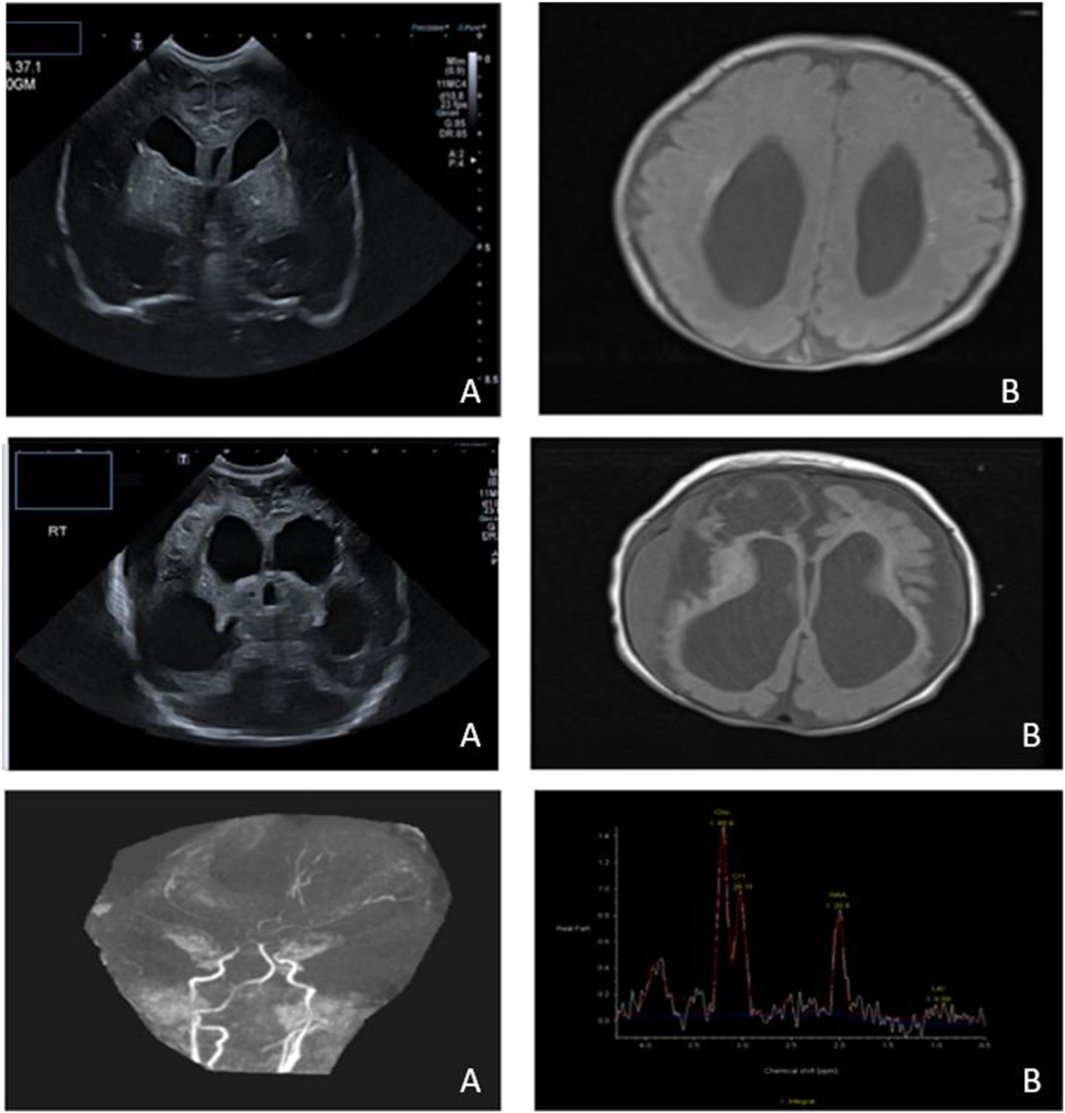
Serial neuroimaging. *Top panel:* (A) cranial ultrasound image on day 1 of life showing ventriculomegaly with colpocephaly (B) MRI brain on day 4 of life: coronal T1 images with dilatation of lateral ventricles with colpocephalic configuration (right > left) with multiple hyperintense foci in periventricular white matter *middle panel:* (A) cranial ultrasound at 3 months showing progressive ex-vacuo dilatation of ventricles and evolving cystic encephalomalacia (B) follow-up MRI at 3 months of life shows significant diffuse volume loss of cerebral white matter with intraparenchymal multicystic changes marked in the right frontal lobe. *Bottom panel:* (A) time of flight MRA study showed severe attenuation of the supra clinoid segments of internal carotid arteries and bilateral M1 and P1 segments, absent flow in the right anterior cerebral artery and small left anterior cerebral artery. (B) MR spectroscopy over the left basal ganglia with low NAA and small lactate peak.
